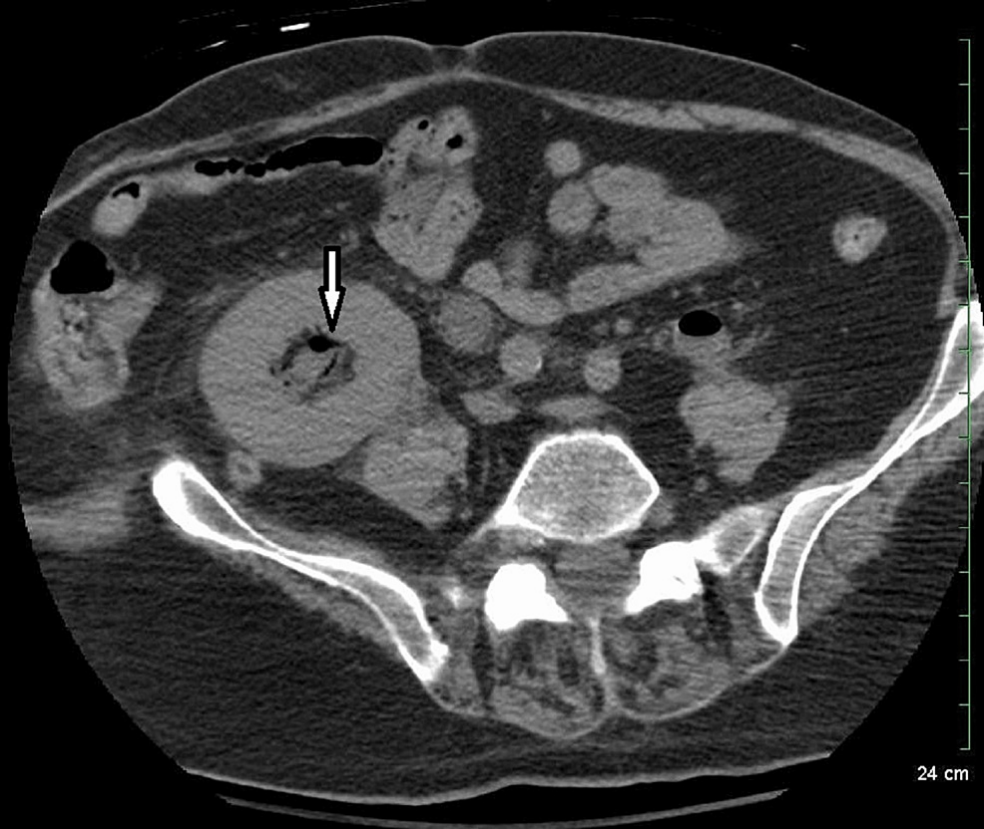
Non-enhanced CT image showing gas in the transplanted kidney pelvis (white arrow).
